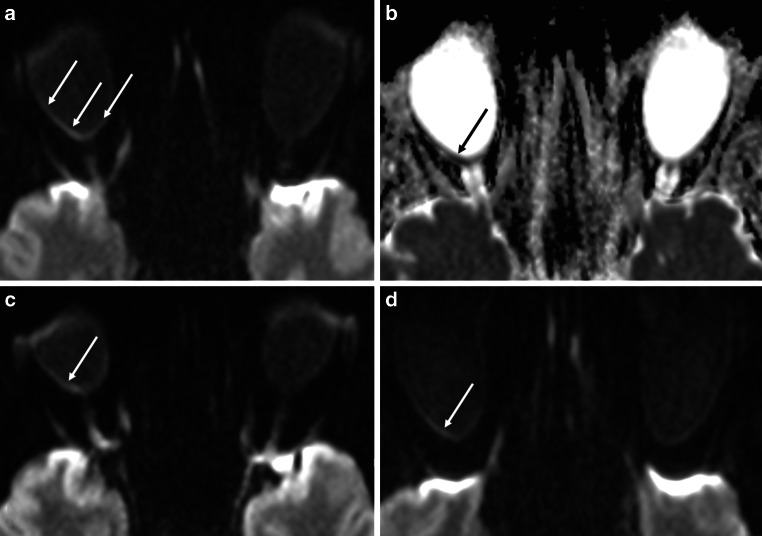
Retinal diffusion restrictions in acute right-sided central retinal artery occlusion in a 56-year-old patient with complete amaurosis. a On standard brain stroke DWI-EPI sequence (b = 1000 s/mm^2^) at 3 T the inner aspect of the posterior half of right globe appears clearly more hyperintense and is better delimitable as well as slightly thickened as compared to the contralateral side (*arrows*). b The ADC map image shows correspondingly reduced diffusion qualitatively (*arrow*). c, d DWI images above and below a showing the same image appearance with clearly positive DWI on the affected right side (*arrow*)
